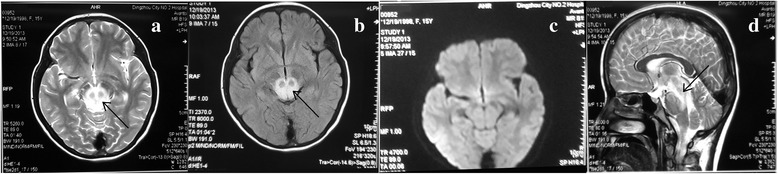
Brain MRI after the symptom onset. T2-weighted imaging (T2WI) and fluid-attenuated inversion-recovery (FLAIR) imaging revealed isolated hyperintense signals in the dorsal part of the pons and the midbrain in axial view (a, b) and sagittal view (d). Diffusion-weighted imaging (DWI), however, showed normal intensity (c)
